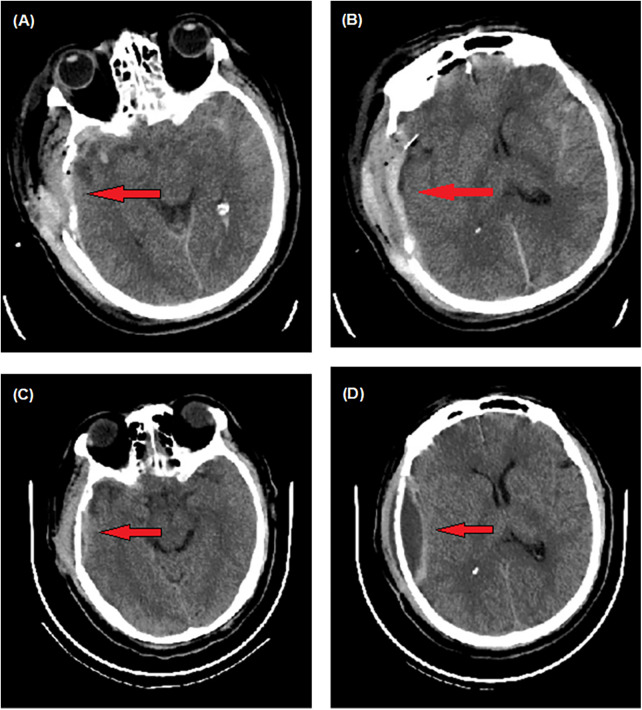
Imaging findings before and after cranioplasty. (A,B) Axial computed tomography (CT) images acquired on March 4, 2025: bilateral intracranial heterogeneous hyperdense and hypodense lesions with compression and narrowing of the right lateral ventricle; hyperdense casting in the left cerebral sulci and cisterns; and increased density of the falx cerebri and tentorium cerebelli. (C,D) Axial CT images acquired on June 23, 2025: heterogeneous fluid-density collection with mixed hyperdense and hypodense components in the surgical region, minimal intracranial and extracranial pneumocephalus; hypodense lesion in the right frontal lobe; and subcutaneous soft tissue swelling in the surgical region.
